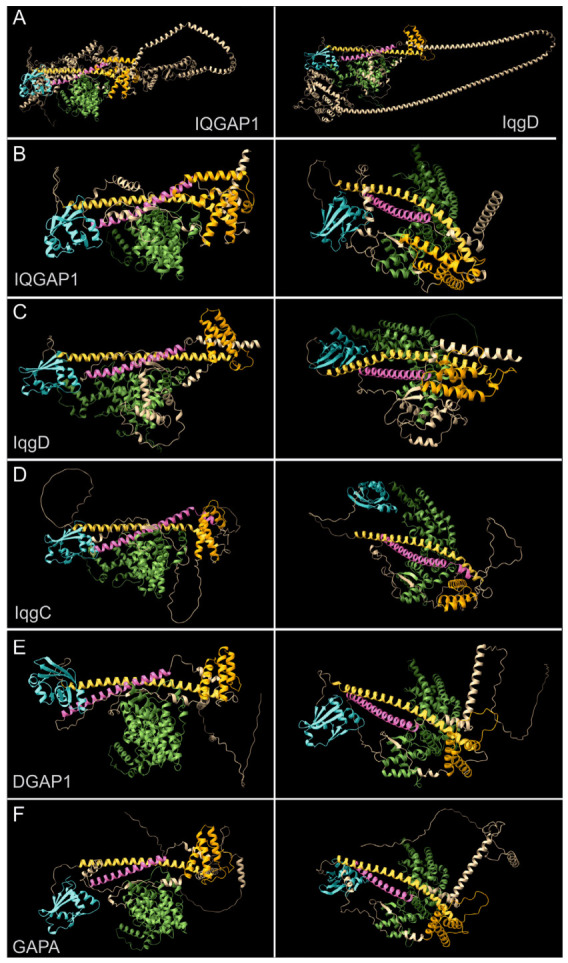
Computationally predicted structures of human IQGAP1 and IQGAP-related proteins from *Dictyostelium discoideum*. (A) Predicted three-dimensional structures of full-length IQGAP1 (left) and IqgD (right) displayed at a reduced scale; (B) C-terminal part of IQGAP1 (amino acid residues 836–1657); (C) C-terminal part of IqgD (amino acid residues 561–1385); (D) full-length IqgC; (E) full-length DGAP1; (F) full-length GAPA. In (B–F), two projections are shown side by side in the left and right columns. Individual structural features are coloured as follows: N-terminal helix (HN) in pink, GAP-related domain (GRD) in green, triplet of short helices within the RasGAP C-terminal (RGCt) domain (RGCt-H3) in orange, C-terminal helix within the RGCt domain (RGCt-HC) in yellow, and the C-terminal domain (CT) in cyan. The remainder of the protein is coloured beige. The intervals of amino acid residues corresponding to each domain are listed in Table 1. Predicted three-dimensional structures were obtained using the AlphaFold web server powered by the AlphaFold 3 model [21]. Visualisation was performed with UCSF ChimeraX [22]. The primary protein sequences were downloaded from the UniProt website [23].
